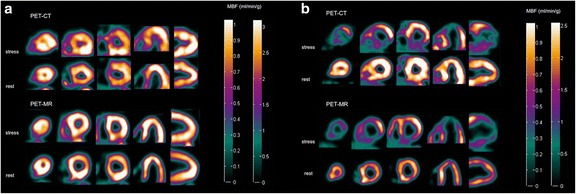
Parametric images of stress and rest MBF from PET-CT and PET-MR std (clinical protocol) with Cardiac VUer software. Images from a patient with normal myocardial perfusion (a) and from a patient with apical, anterior and inferior ischemia (b). The colour scale is absolute (i.e. in mL/g/min) and identical for the PET-CT and the PET-MR images for each patient
